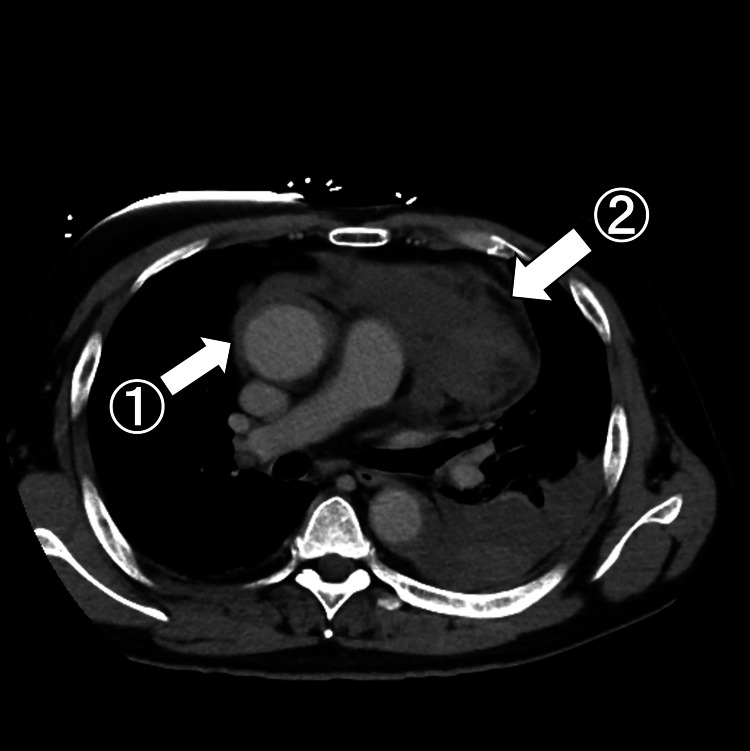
Chest contrast CT Aortic dissection of Stanford type A is seen ①. After sternal compression, a large amount of blood is seen in the anterior aspect of the pulmonary artery ②.
